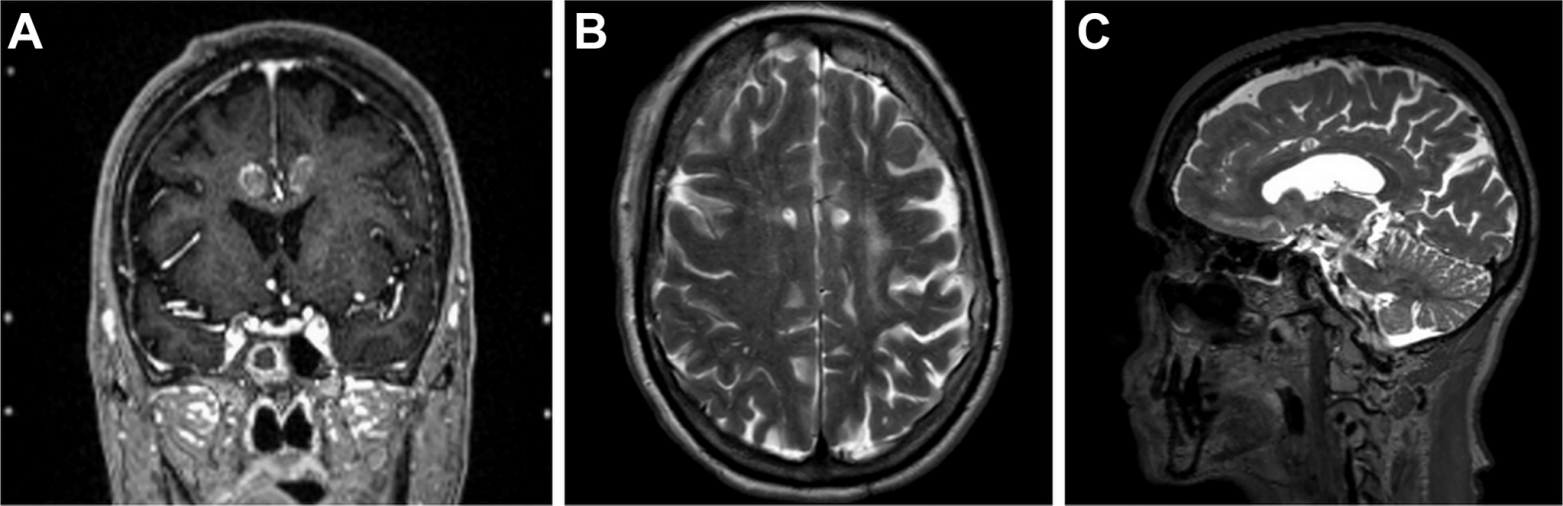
Magnetic resonance images of bilateral anterior cingulotomy in the coronal plane (A) intraoperative, and in the axial (B) and sagittal plane (C) one-year postoperative.
